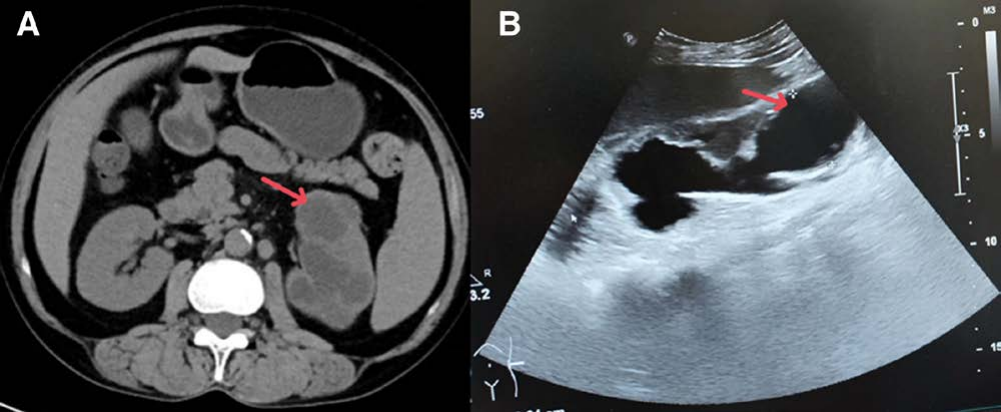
(A) Initial abdominal computed tomography scan showed low-density shadows in the left kidney. (B) At the intensive care unit admission, ultrasound showed left hydronephrosis, parenchymal atrophy, and hydroureter.
